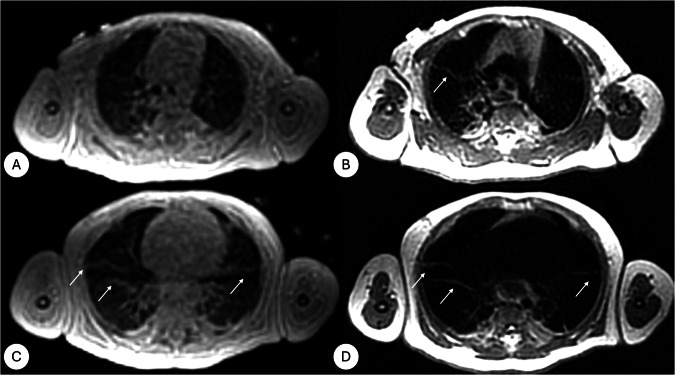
Axial ZTE (A, C) and axial T2-w PROPELLER (B, D) sequences of patient 2 at corresponding locations, showing hyperintense regions (linear opacities, arrows). Note that on ZTE images (A, C), the hyperintense regions are difficult to identify due to a low SNR ratio. The MERGE score showed an average of 14.5% diseased lung tissue, with hyperintense regions comprising 12.1% of the total lung volume. No bronchopathy was seen in this patient. ZTE, zero echo time; PROPELLER, periodically rotated overlapping parallel lines with enhanced reconstruction; SNR, signal-to-noise ratio; MERGE, the bronchopulMonary RottErdam MRI morpholoGy scorE
